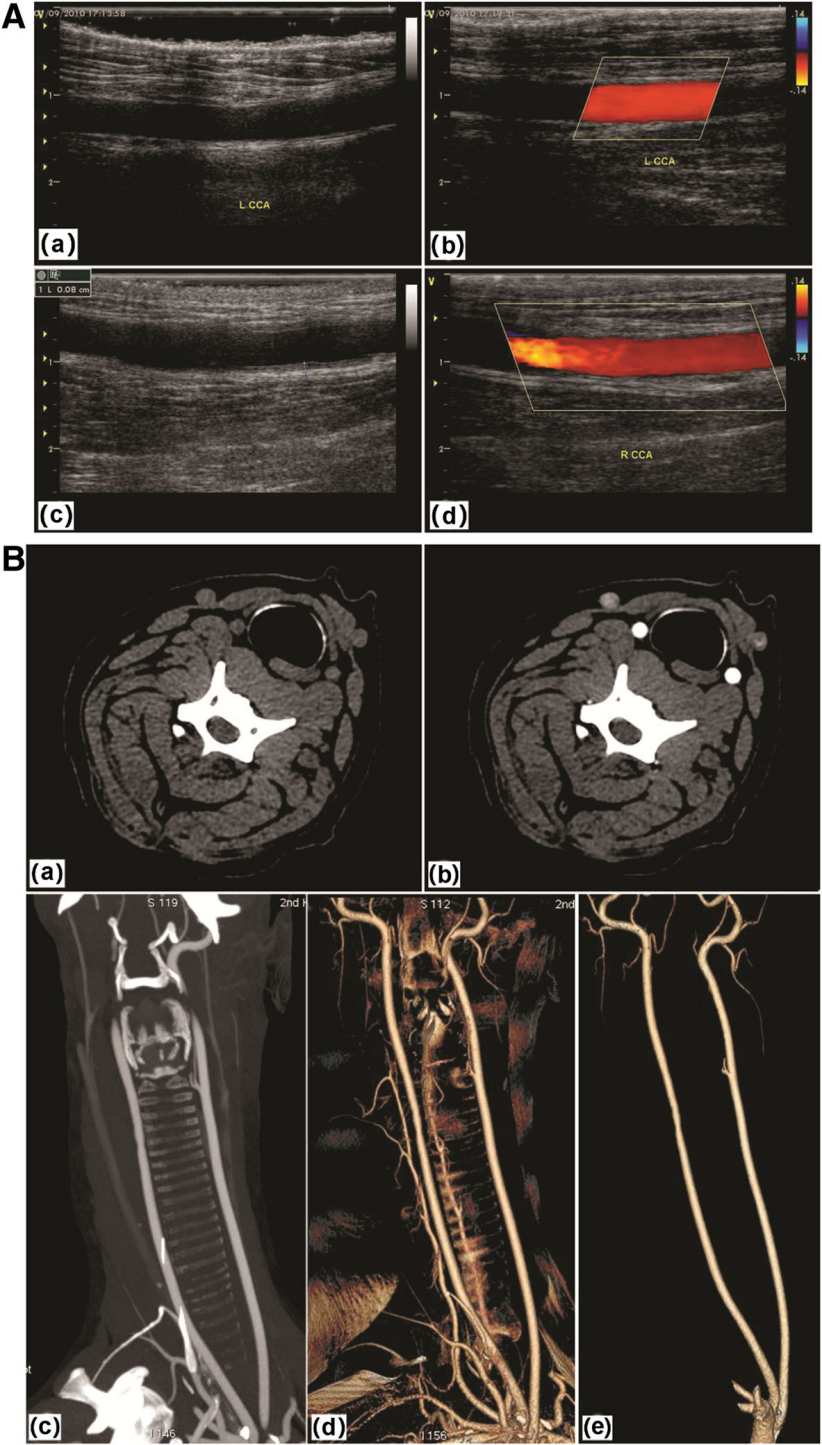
Color Doppler ultrasonography and three-dimensional CT detection after the implantation of tissue-engineered vascular grafts into canine common carotid arteries. A: a and b, Color Doppler ultrasonography at 3 months post-implantation; c and d, 6 months post-implantation. B, three-dimensional CT detection at 6 months post-implantation. a and b are horizontal images; c is a coronal plane image; d and e are three-dimensional images
